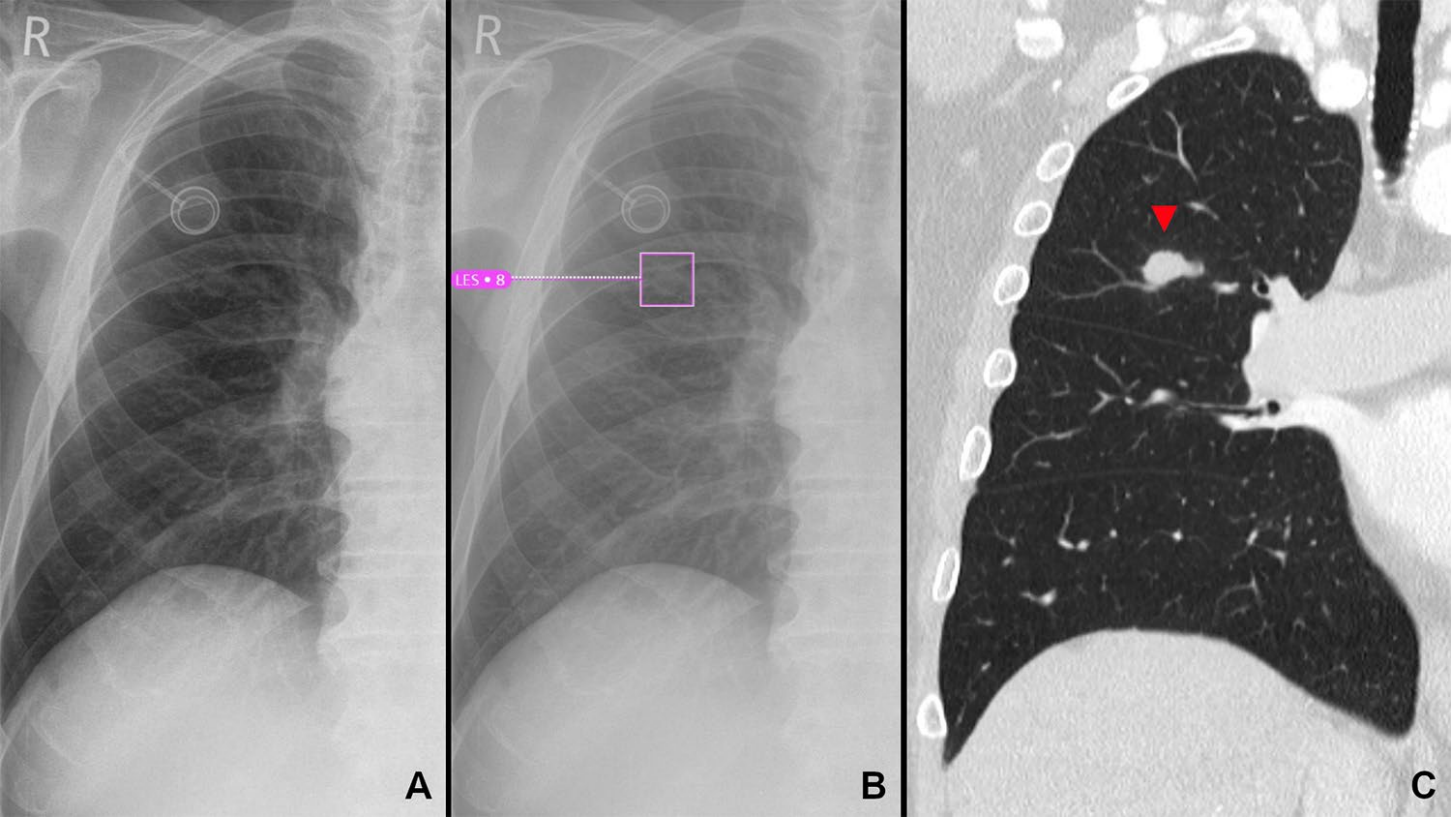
Example of a lung lesion detected by the AI Rad Companion Chest X-ray (A, B) that was confirmed by a CT scan of the chest (C): 74 year old, male patient diagnosed with a carcinoma of the tongue 2 years ago.
